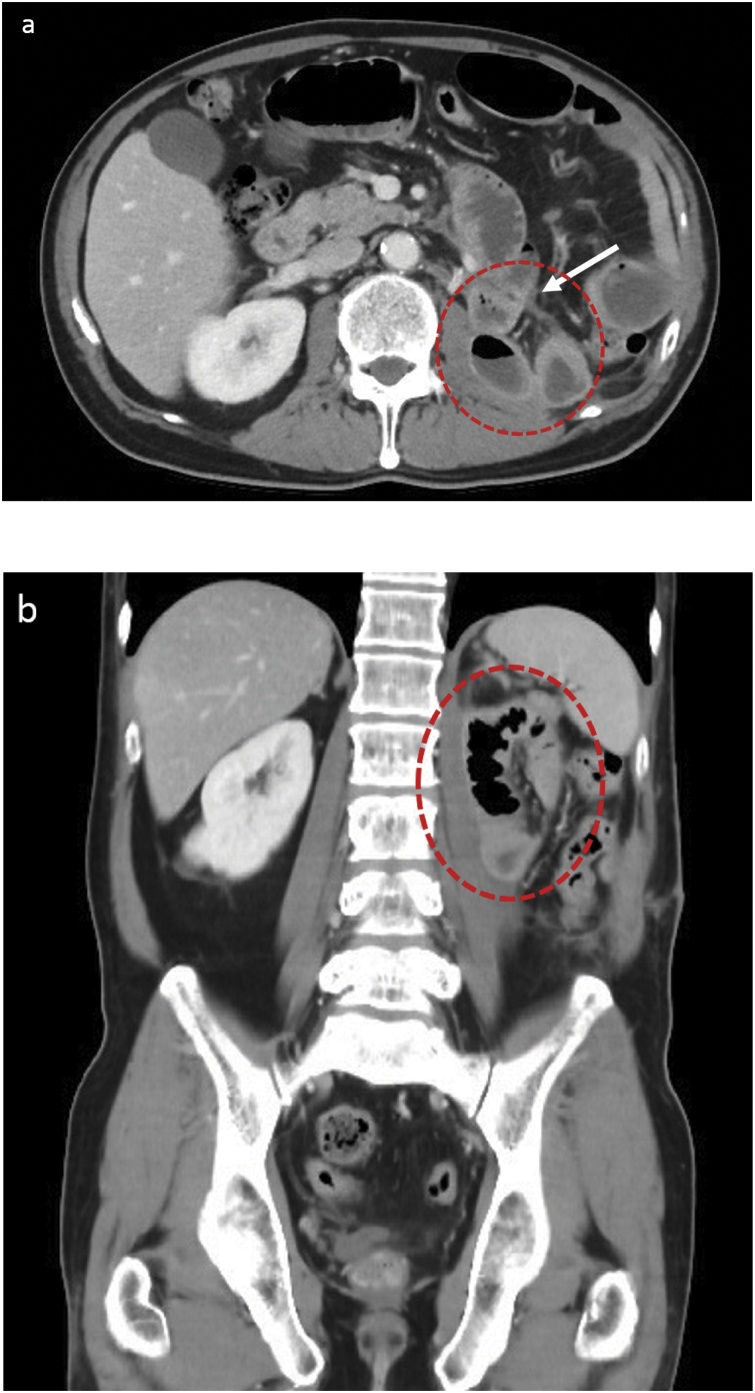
Enhanced computed tomography (CT) image showing the incarcerated small intestine in left retroperitoneal space. (dotted red circle: incarcerated small intestine, white arrow: the hernia orifice, a: horizontal image, b: coronal image).
